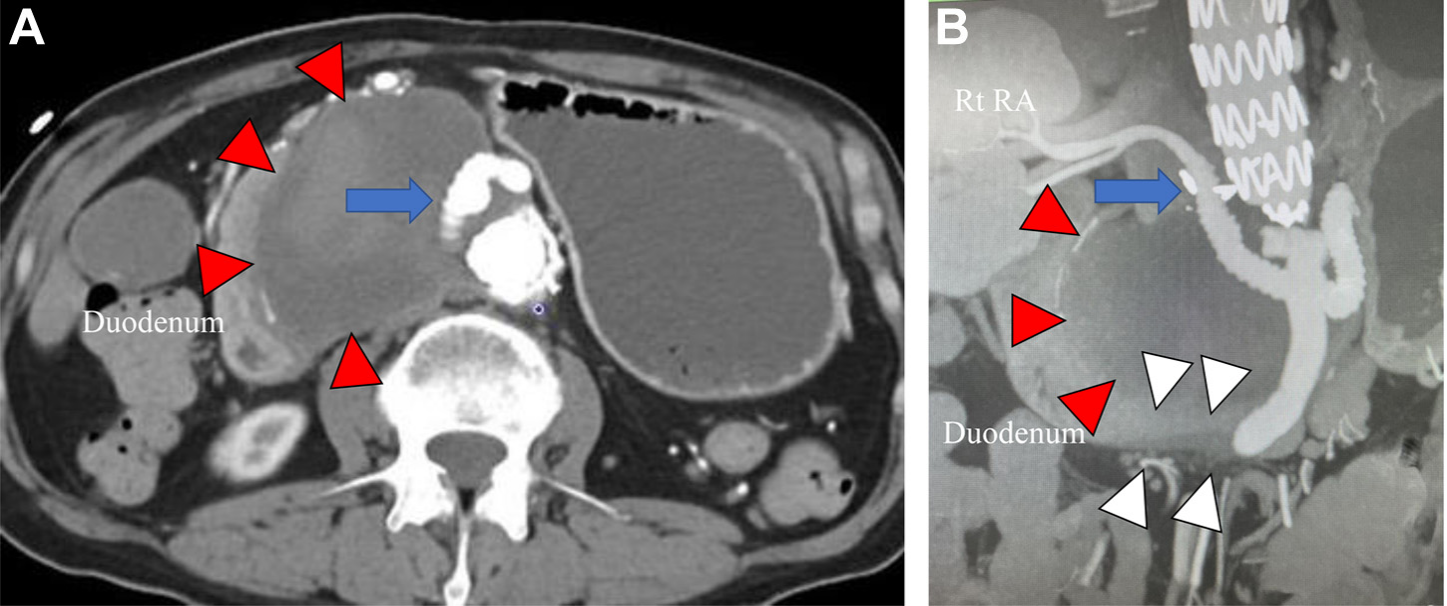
Contrast enhanced computed tomography (CT) images. A, Axial view. B, Coronal view. Contrast-enhanced CT revealed dilated regions in the stomach and duodenum and an abdominal low density mass (*red arrow*) compressing the third position (*white arrow*). The mass was ∼95 mm in size and located around the bypass graft to the right (*Rt*) renal artery (RA; *blue arrow*). No extravasation of contrast media was noted in the area of the mass.
